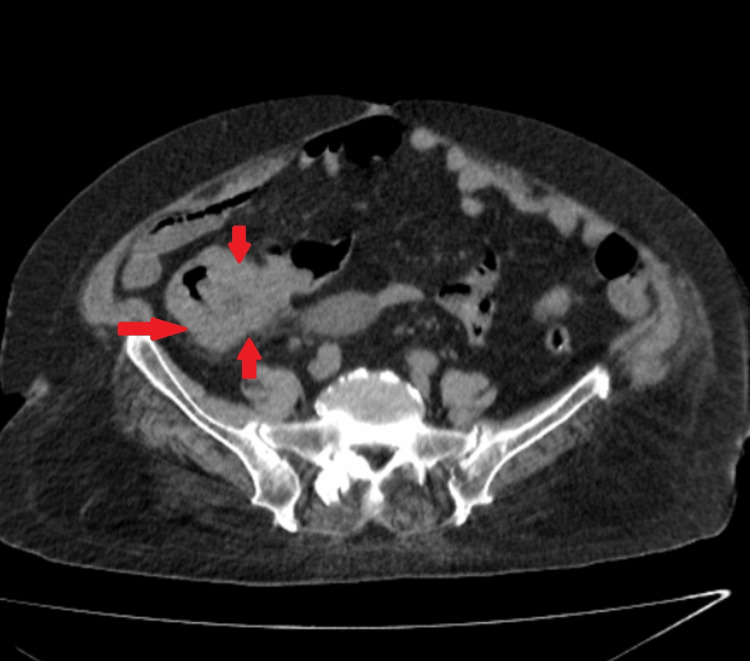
Axial contrast-enhanced CT image showing suspicious cecal wall thickening Axial contrast-enhanced CT image showing irregular circumferential thickening of the cecal wall, suspicious for an underlying malignant lesion. The red arrow indicates the abnormal cecal wall thickening. No bowel obstruction was identified on CT. CT: computed tomography
